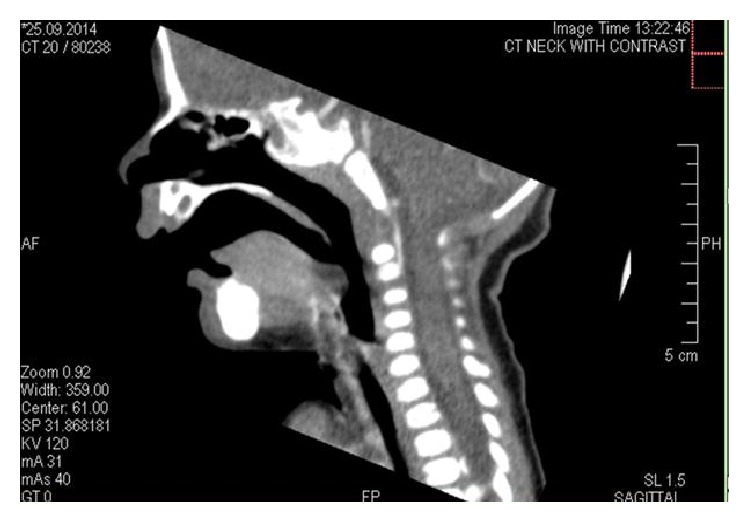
Sagittal CT scan showing a retroglossal fairly defined rounded nonenhancing hypodense cystic lesion measuring about 9 mm, seen projecting into oropharynx.
